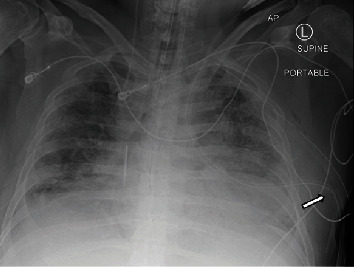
Last available preintervention chest X-ray showing kinking of chest tube #1 in the subcutaneous tissue (arrow).
